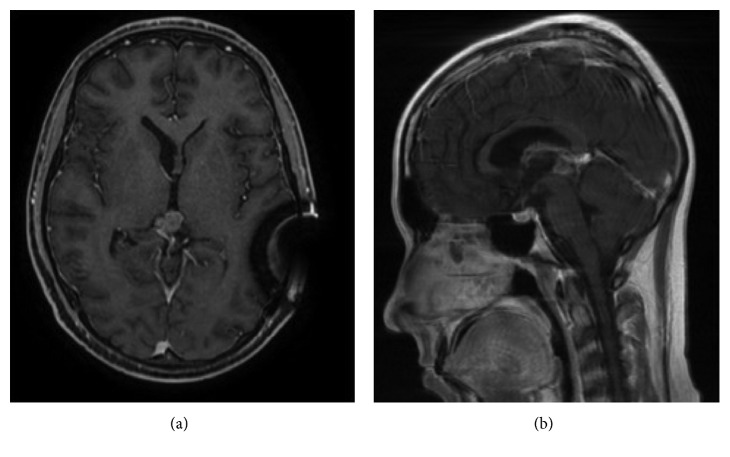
MRI after chemotherapy shows residual tumor mass. For this lesion, the patient underwent stereotactic radiosurgery.
